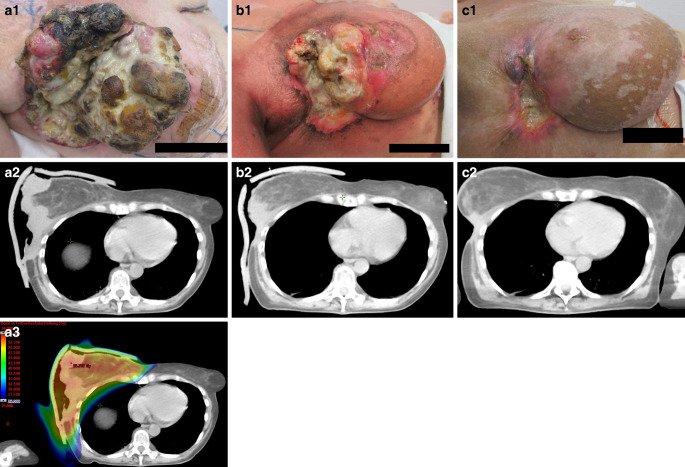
a–c Example of a patient with a malignant wound responding well to RT (tumor mass reduction and improvement in wound conditions) and achieving the treatment goal (Breast carcinoma, invasive carcinoma without special type, grade 3, triple negative, T4b N1 M1, combined radio-chemotherapy with vinorelbine). (*a1*) Wound documentation before RCT, (*a2*) axial slice of the pre-treatment RT planning CT scan, (*a3*) corresponding VMAT radiotherapy plan, dose color wash ranging from 25.0 Gy (blue, lowest value) to maximum dose (55.757 Gy, red, base plan) on this plane, (*b1–2*) wound documentation and CT scan at the end of RCT (total dose: 66.6 Gy/1.8 Gy/fraction) and (*c1–2*) 2 months after RCT showing treatment response
